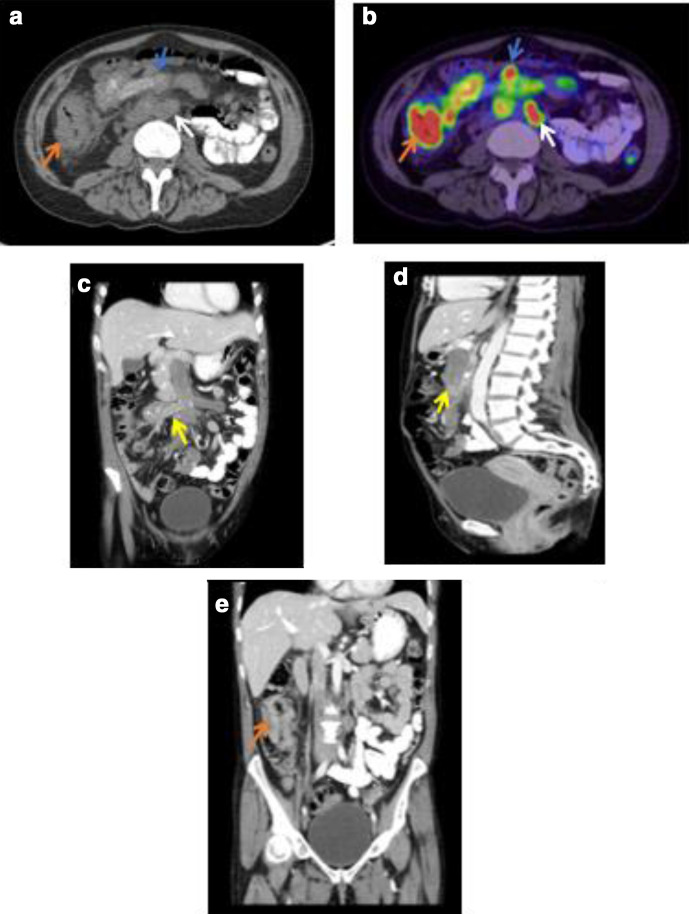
(a-e) Staging (a) Axial CT and (b) FDG-PET CT images showing intensely FDG-PET avid primary caecal tumour (red arrow) and partially calcified tumour thrombus in the SMV (blue arrows) extending into tributaries. Para-aortic nodal metastases are noted (white arrows). (c) Coronal and (d) Sagittal CT images confirm the extensive venous thrombus (yellow arrows) and (e) shows the caecal mass (red arrow). Bland thrombus is most likely to be on the downstream tip of tumour thrombus. FDG, Fludeoxyglucose; PET, Positron emission tomography; SMV, Superior mesenteric vein.
